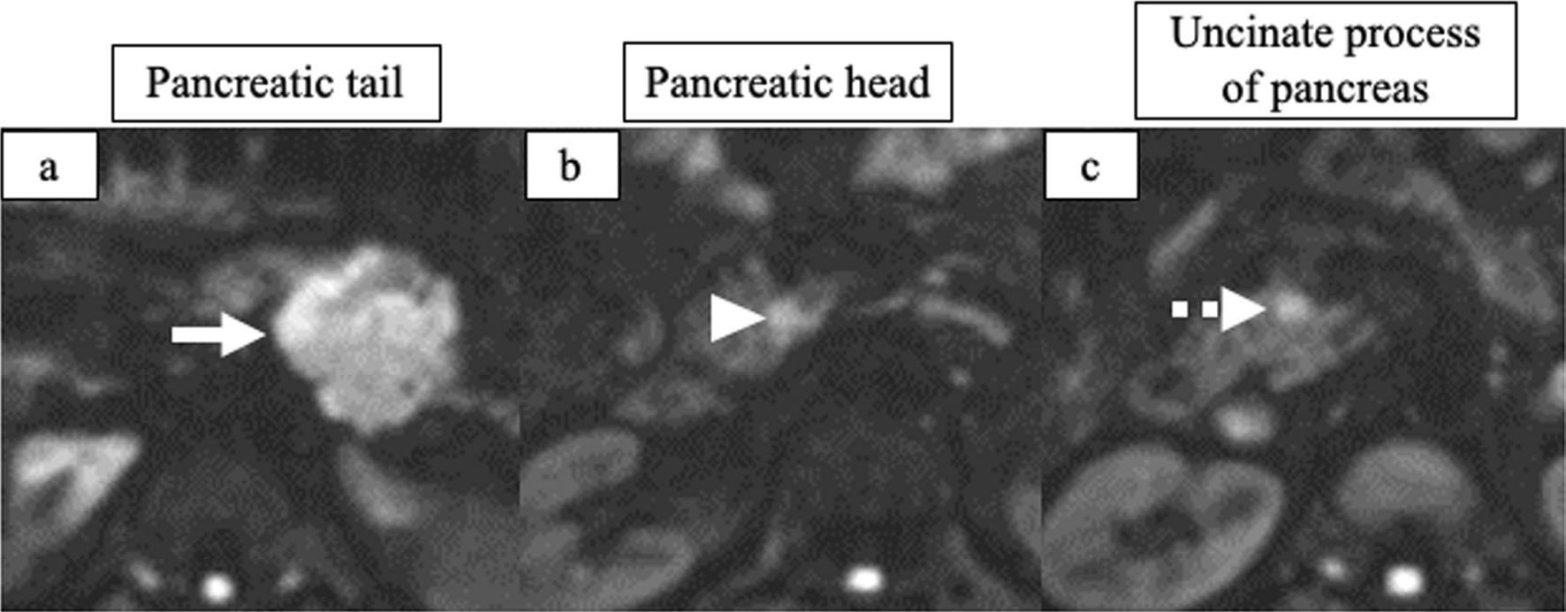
MRI diffusion-weighted imaging. a The tumor located in the pancreatic tail is seen (white arrow). b The tumor located in the pancreatic head is seen (white arrowhead). c The tumor located in the uncinate process of the pancreas is seen (dotted white arrow). *MRI* magnetic resonance imaging
